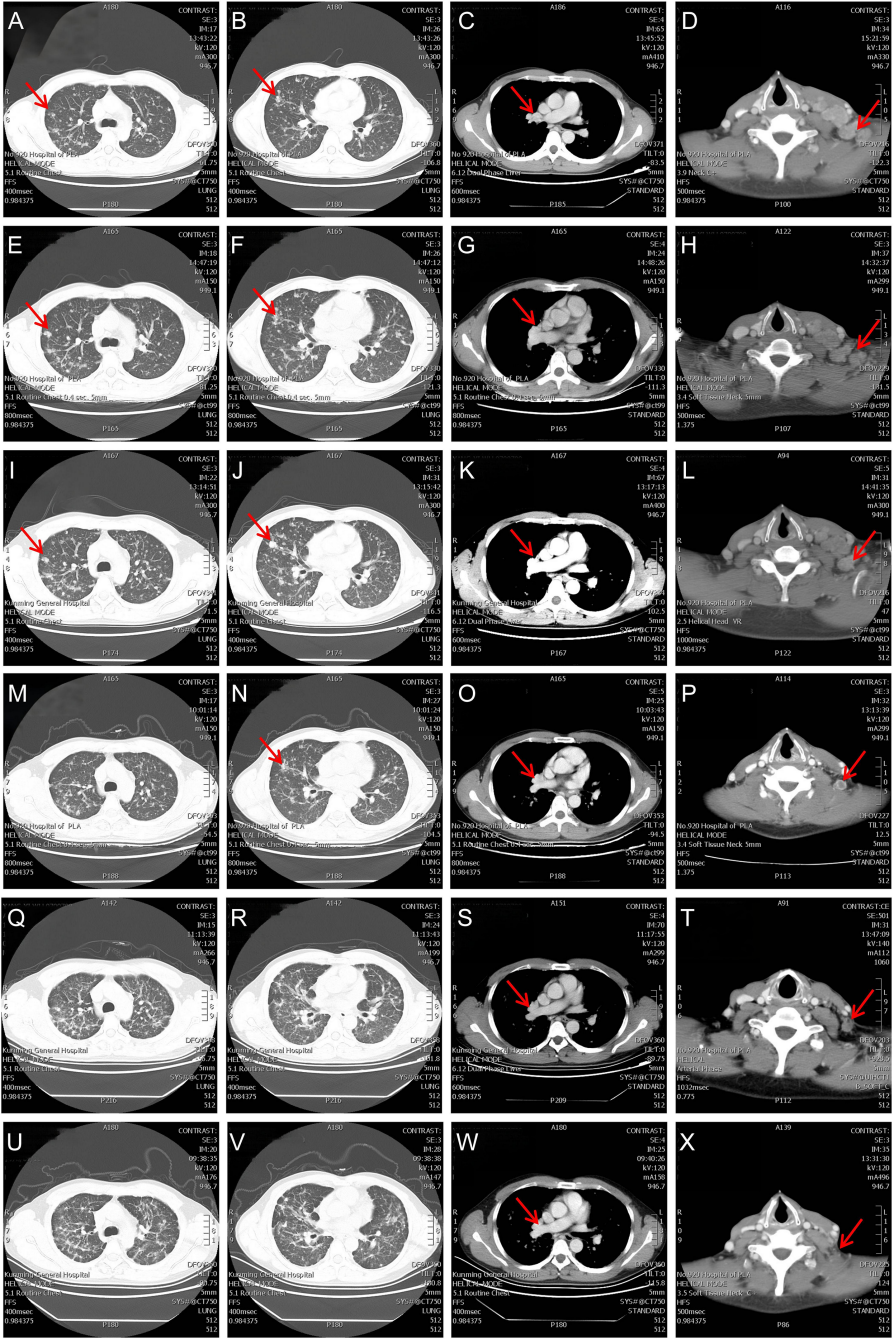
Longitudinal CT evaluation of pulmonary sarcomatoid carcinoma across therapeutic phases. (A-D) Baseline (2019-07-17): Left supraclavicular lymphadenopathy (29 mm), right hilar adenopathy (14 mm), and multifocal pulmonary metastases (arrows). (E-H) After 2×TP chemotherapy cycles (2021-09-17): Progressive enlargement of bilateral lung metastases (RECIST-defined progression). (I-L) After 2×PC chemotherapy cycles (2019-11-11): Continued progression of pulmonary lesions. (M-P) After 8×anlotinib cycles (2020-04-07): Significant regression of left supraclavicular nodal and pulmonary metastases. (Q-T) Post-31-month anlotinib (2022-06-17): Left supraclavicular nodal progression (PFS1 = 31 months). (U-X) After camrelizumab-anlotinib combination (2025-04-28): Stable disease (SD) with sustained remission (PFS2 = 37 months).
